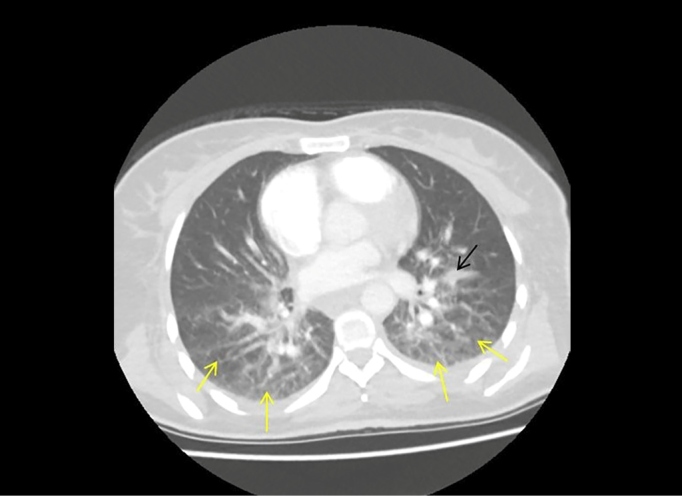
Diffuse complete-incomplete consolidation areas and increased reticulonodular thickness (yellow arrows) in the lower lobes of the bilateral lungs in axial thoracic computed tomography imaging. Fluid collection consistent with fissuritis visible in the left major fissure (black arrow)
